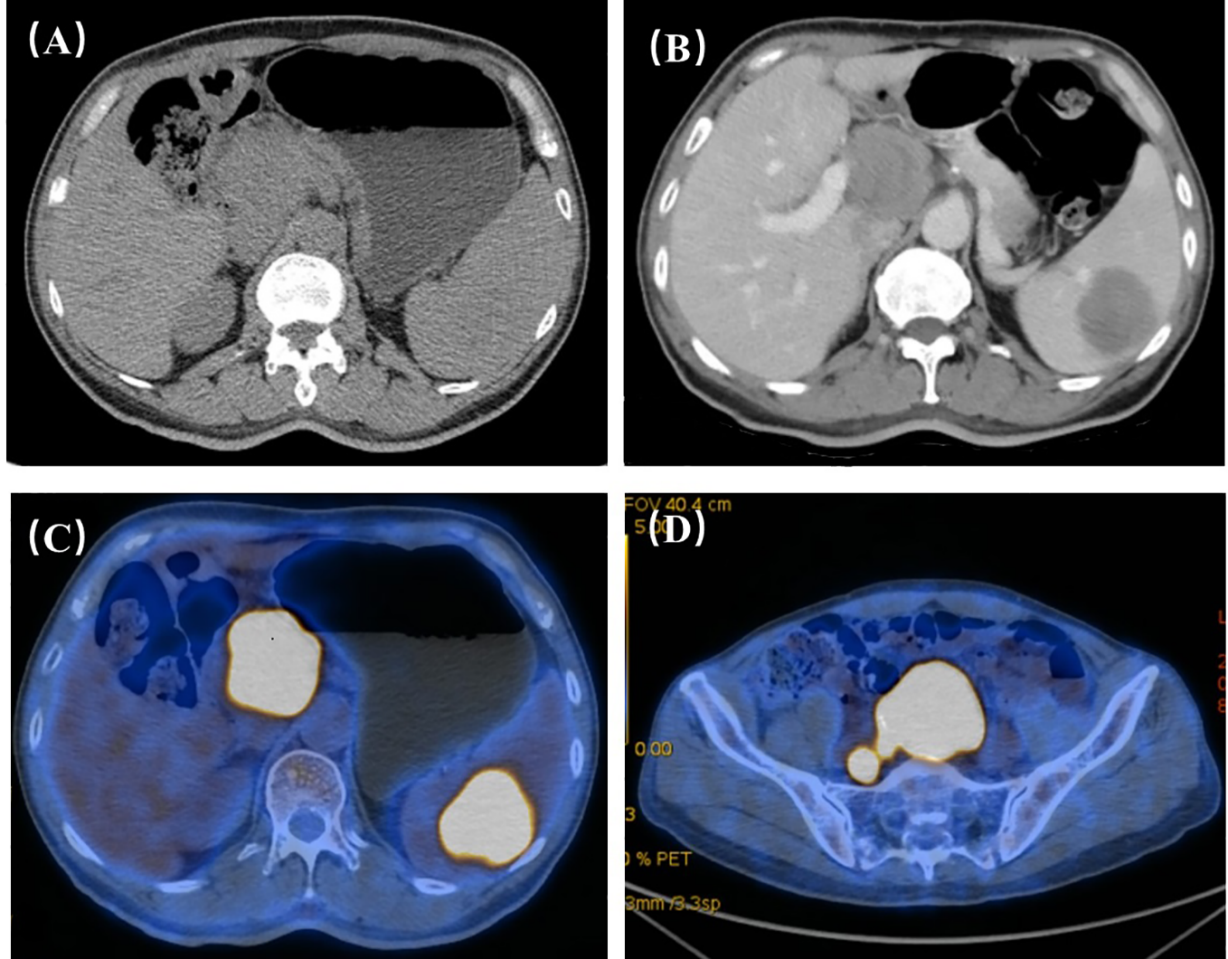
(A) Through plain scan of the upper abdomen, it clearly showed the masses in the hilar region, but the masses in the spleen were not obvious. (B) Through contrast-enhanced CT, it could show the masses in the hilar region and splenic region, and the masses showed progressive and uneven enhancement. The hilar region nodules: plain scan 30HU arterial phase, 40HU portal vein phase, 52HU delayed phase, 65HU; spleen nodules: plain scan 35HU arterial phase, 46HU portal vein phase, 52HU delayed phase, 63HU; upper abdominal enhancement suggested: Further examination is recommended for hilar and splenic masses. (C, D) FDG metabolism of hilar mass, splenic mass and presacral mass on PET-CT increased, and finally PET-CT suggested that the three were homologous and had a high possibility of lymphoma.
